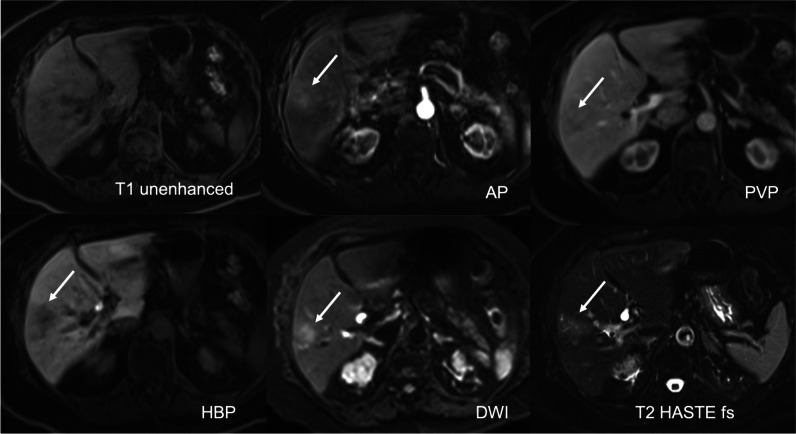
Severe mixed AP artifacts, score 4, optimal timing, uncertain lesion visibility, score 2: Multiple bright and dark lines parallel to the liver edge represent mild TA (score 2). Misregistration of the right kidneys and blurring at liver and kidney boundaries indicate severe TSM (score 4): This 94-year-old woman with Merkel cell carcinoma of the left cheek was sent for MRI to rule out liver metastases. A T2 mildly hyperintense lesion in liver segment V shows weak enhancement on the standard AP with washout in the hepatobiliary phase. DWI shows some restrictions centrally. A biopsy confirmed that this was a hypervascular metastasis. The diagnosis was uncertain due to the poor lesion visibility
